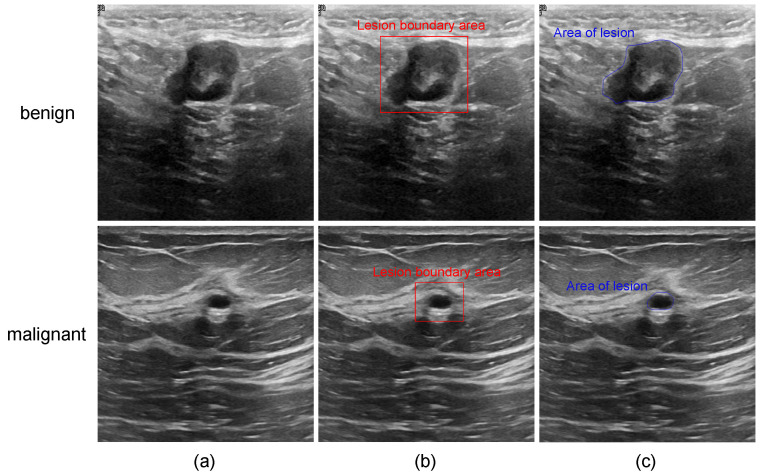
Some examples of breast ultrasound image: (a) original images of benign and malignant lesions; (b) images with the boundary of the lesion area; and (c) images with the specific lesion area.
